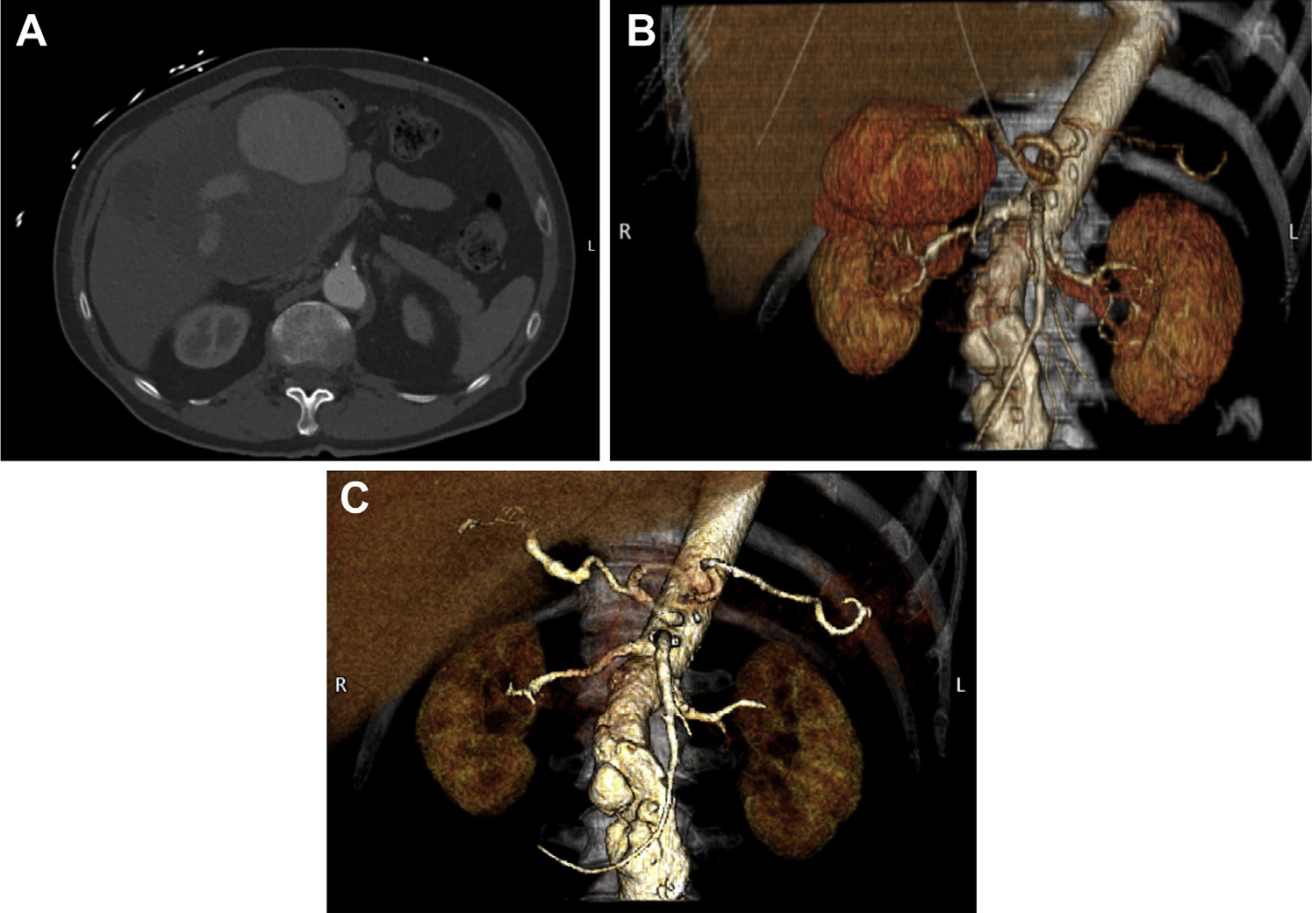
A, Abdominal computed tomography (CTa)scan showing intact giant true hepatic artery aneurysm compressing the liver hilum. B, Three-dimensional reconstruction showing the hepatic artery aneurysm. C, Three-dimensional reconstruction after repair.
